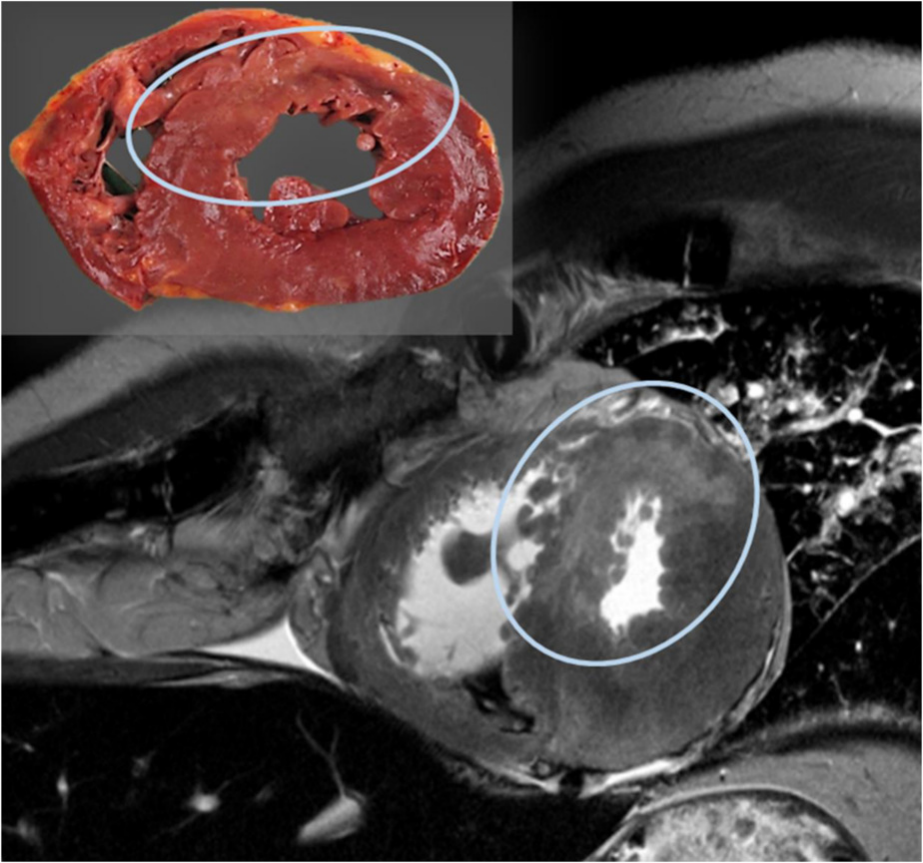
Post-mortem MRI examination of the heart shows a hyper-intense (bright) region in the septum and parts of the anterior wall of the left ventricle on the T2-weighted image, indicating myocardial edema; the corresponding autopsy slice is located in the upper left.
